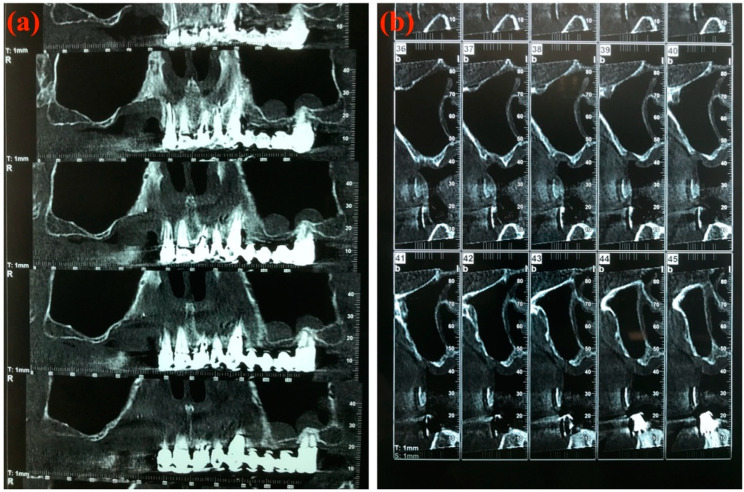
Computed tomography Denta Scan at 6 months of follow-up. (a) Panorex view; (b) Cross-sectional view.
